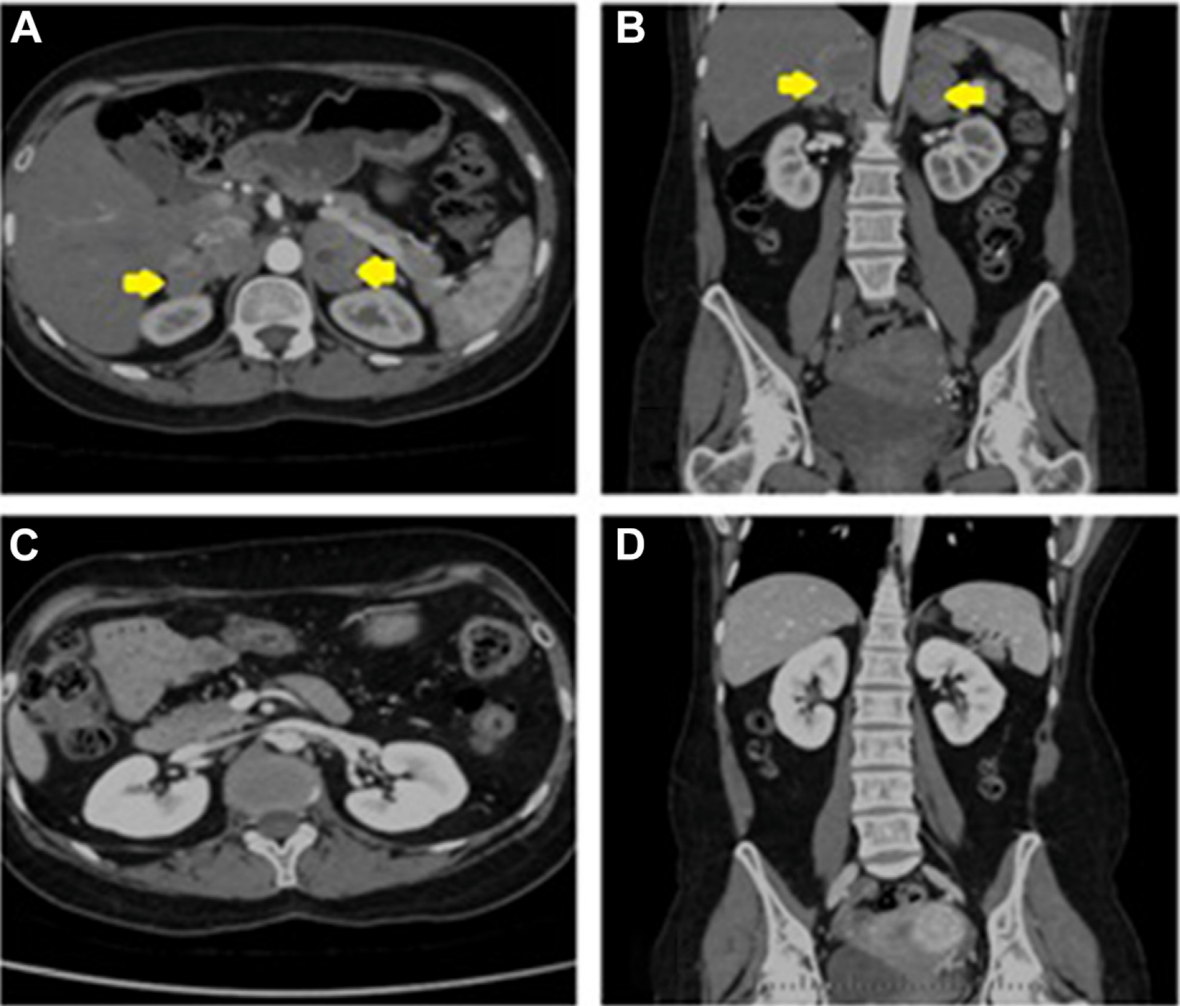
Axial and coronal computed tomography (CT) images demonstrating bilateral adrenal masses before and after adrenalectomy. *A*, Axial view preoperative CT scan. *B*, Coronal view preoperative CT scan (preoperative CT image: yellow arrows showing presence of bilateral pheochromocytoma). *C*, Axial view postoperative scan. *D*, Coronal view postoperative CT scan (postoperative CT images showing absence of bilateral pheochromocytoma following surgical resection).
